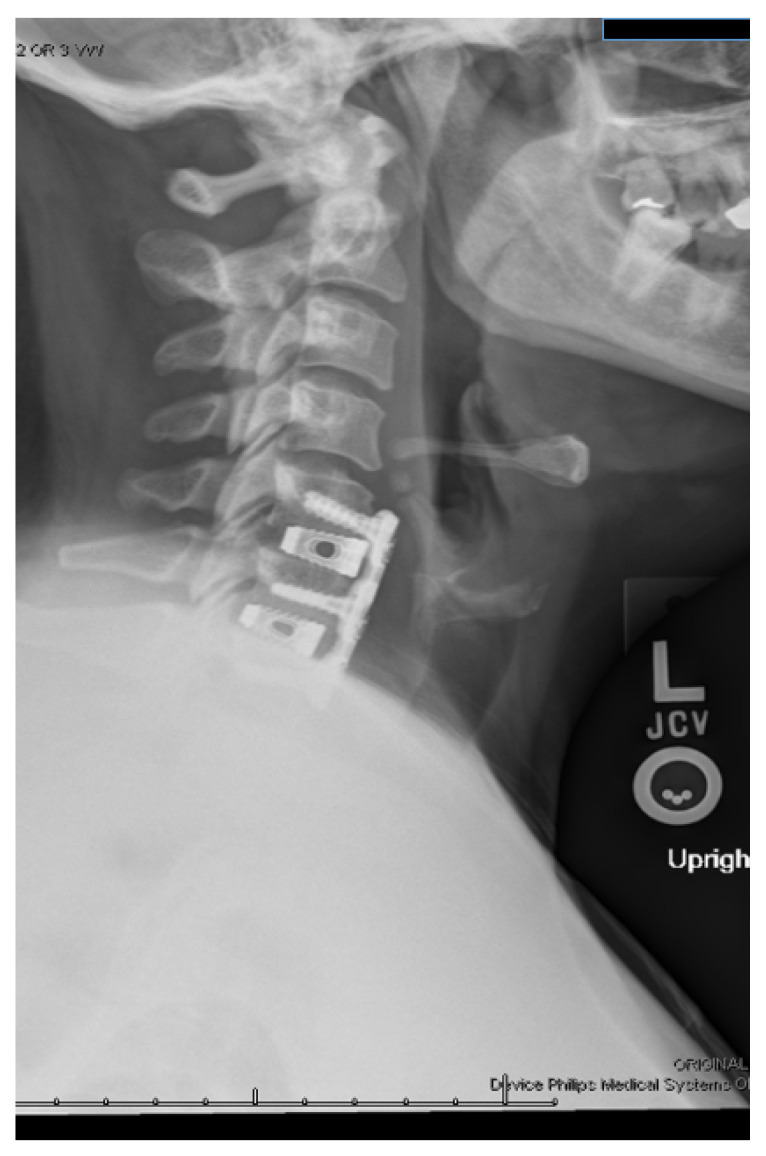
Cervical spine X-ray demonstrating a C5–C7 anterior discectomy and fusion using titanium interbodies with plate fixation.
